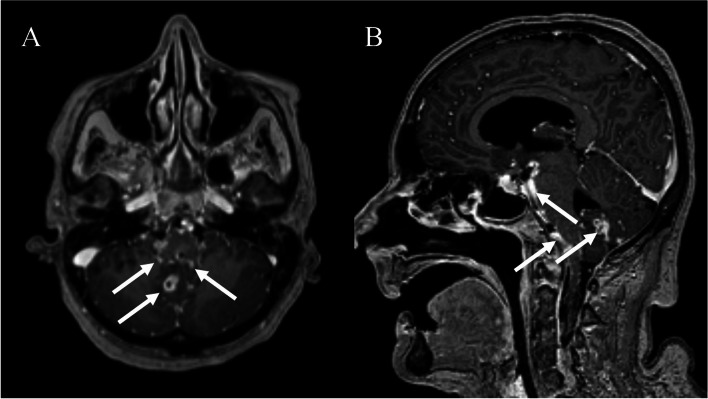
Contrast enhanced, T1-weighted MRI scan 35 days into treatment. A Axial section, showing several ring-shaped contrast enhancements (arrows) with a maximum diameter of 12 mm left cerebellar, right cerebellar and centrally in the cerebellar vermis. B Sagittal section showing leptomeningeal contrast enhancement (arrows), particularly in the cerebellum, medulla oblongata, and pons
